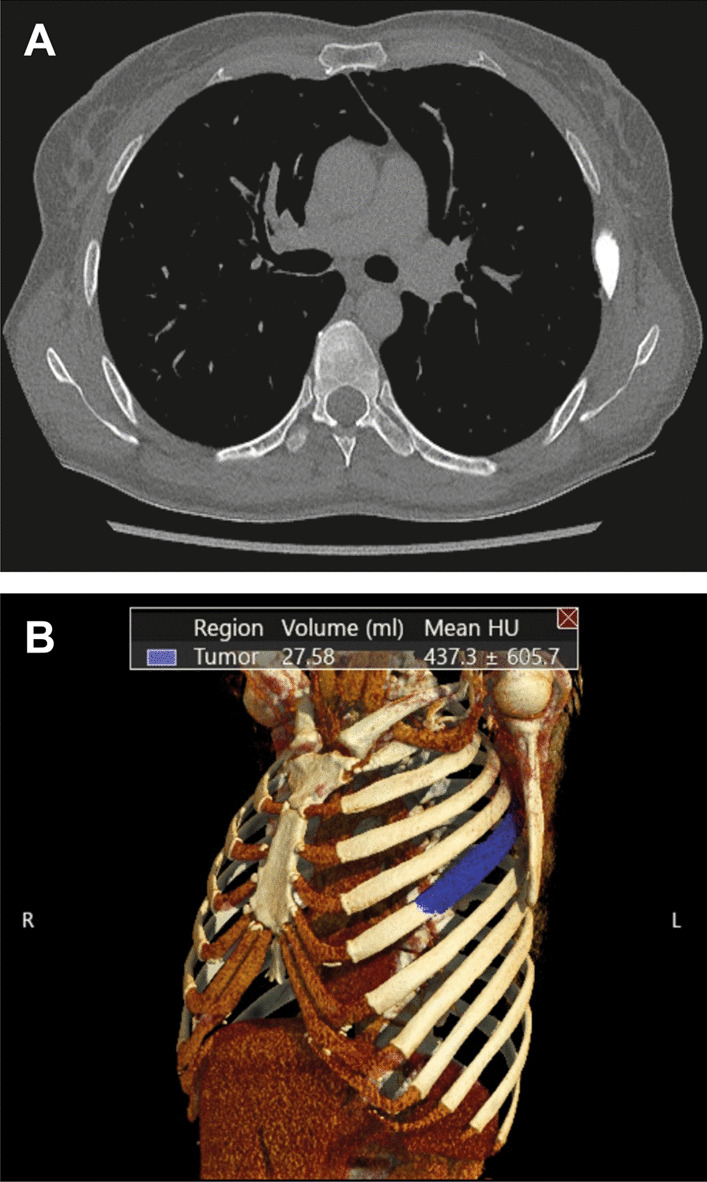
A The axial CT scan vividly displays the expansion of the 5th rib metastasis in NSCLC. B In the CT reconstruction of the NSCLC metastasis, the tumor is delineated in blue, and its volume is quantified (27.58 ml)
